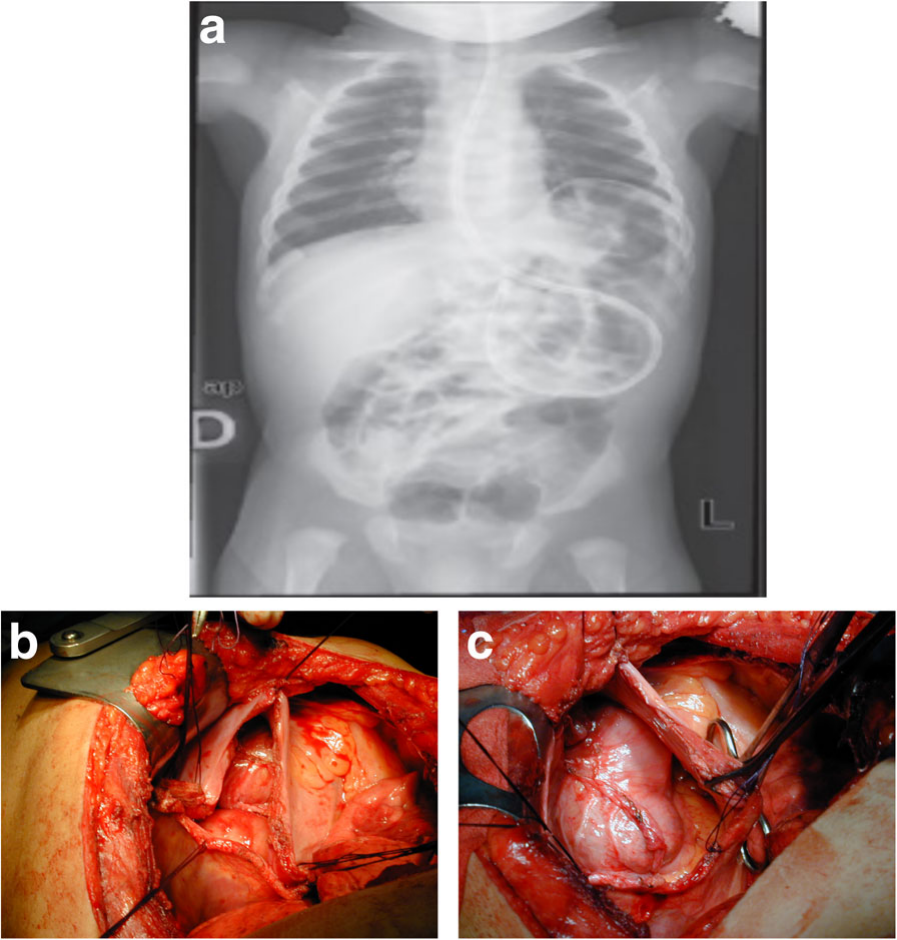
a X-ray shows herniated stomach into the chest. b Thoracotomy shows large diaphragmatic defect. c Repair of defect
